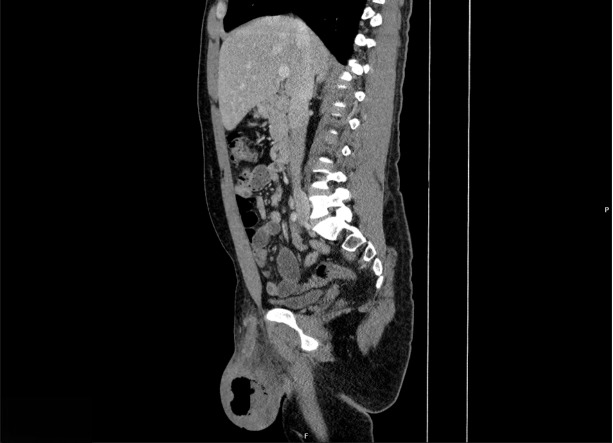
Computed tomography (CT) (sagittal plane) of the abdomen and pelvis confirms the presence of gas and debris in the right side of the scrotum, including within the testicle and epididymis.
